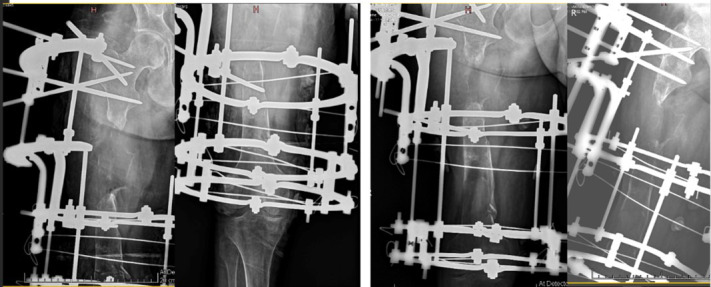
Right femur radiographs during Ilizarov-assisted distraction osteogenesis: immediate postoperative AP and lateral views (left) and 70 days follow-up AP and lateral views showing poor regenerate formation at the corticotomy site (right).
